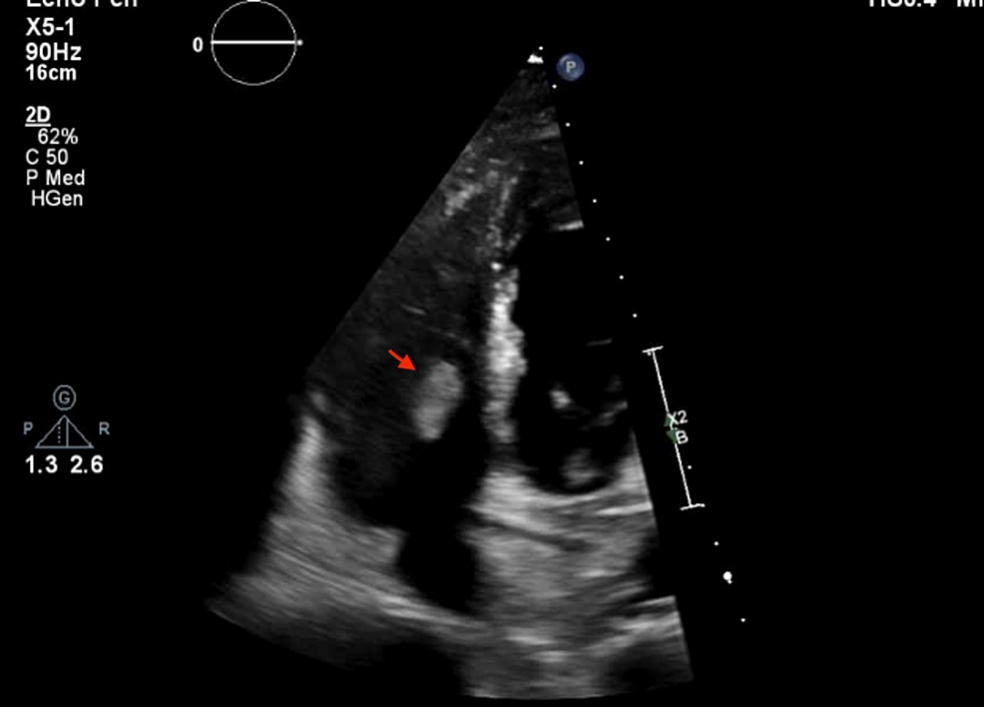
2D transthoracic echocardiography Mobile echogenic structure in the right atrium with trace tricuspid regurgitation.
